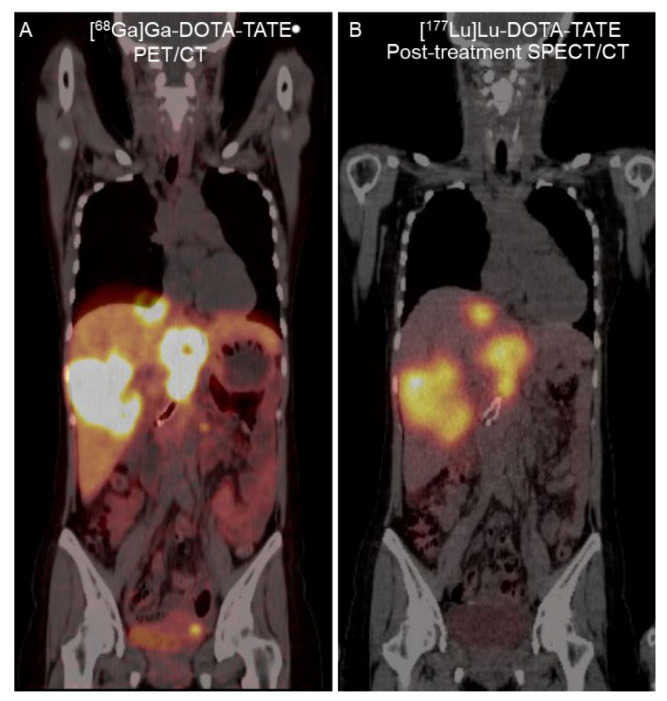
A 32-year-old woman with metastatic pancreatic NET. (A) Coronal fused [^68^Ga]Ga-DOTA-TATE PET/CT image revealed SSTR-positive liver metastasis. (B) Twenty-four-hour [^177^Lu]Lu-DOTA-TATE post-treatment fused single-photon emission computed tomography (SPECT)/CT image revealed similar [^177^Lu]Lu-DOTA-TATE uptake in the liver metastasis.
